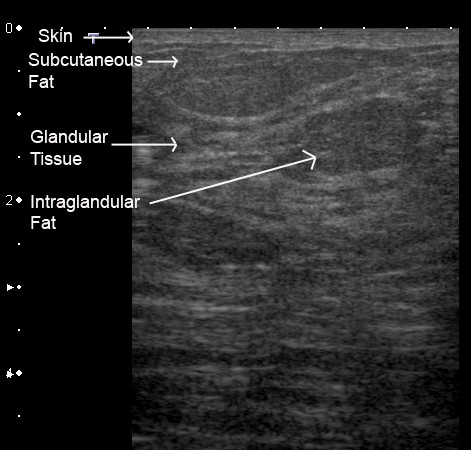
Ultrasound image of the lactating breast. The skin is displayed anteriorly as an echogenic line. Glandular tissue is hyperechoic and the fat more hypoechoic compared to the glandular tissue. Note that there is moderate amount of fat within the parenchyma of the breast.
